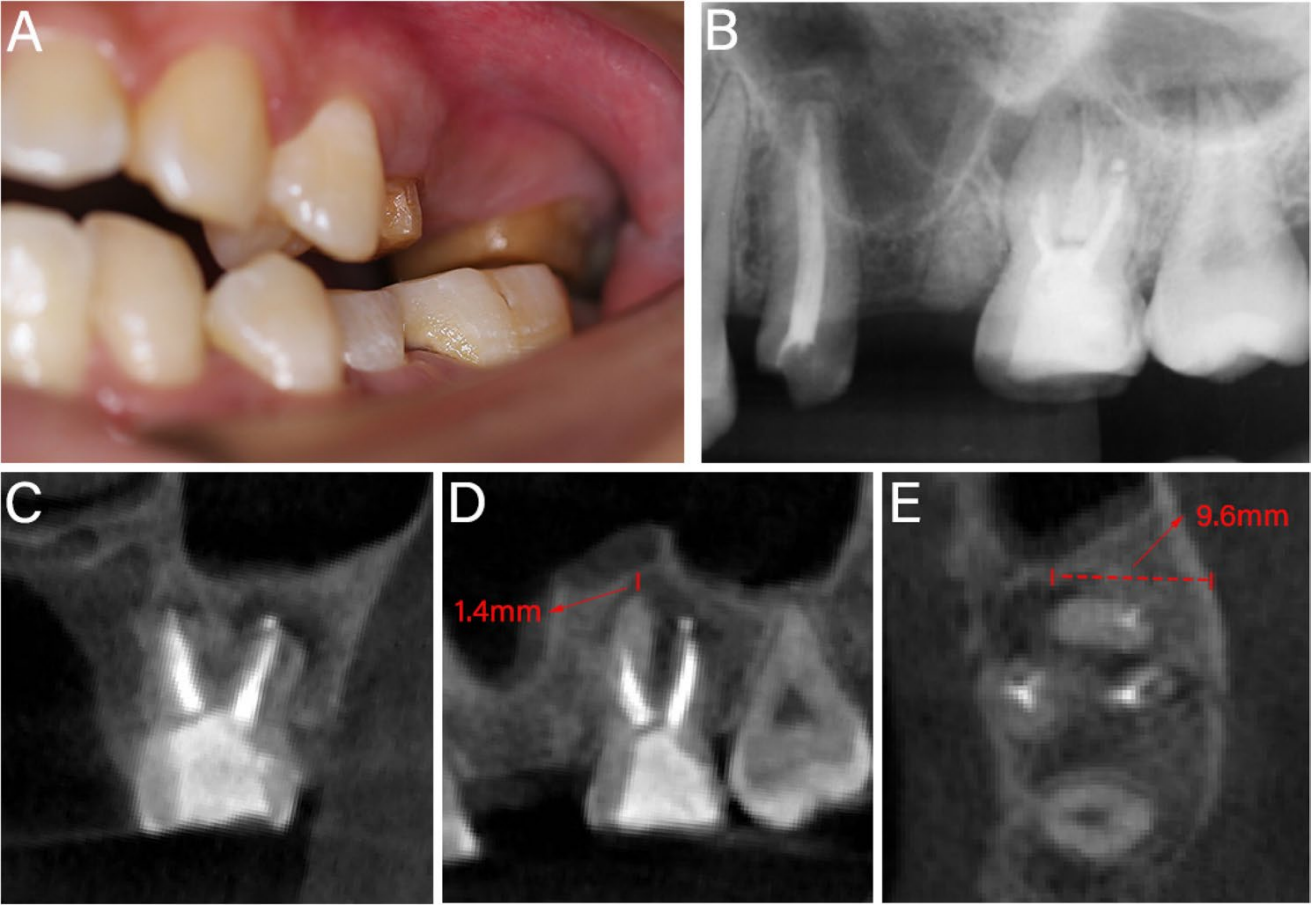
Preoperative examination. A Preoperative photograph. B Preoperative periapical radiograph. C Coronal view of preoperative CBCT. D Sagittal view of preoperative CBCT, showing MB apex distance 1.4 mm from the maxillary sinus. E Axial view of preoperative CBCT, showing intact buccal cortical plate and the resection depth was 9.6 mm from the MB root's lingual surface to the cortical bone
